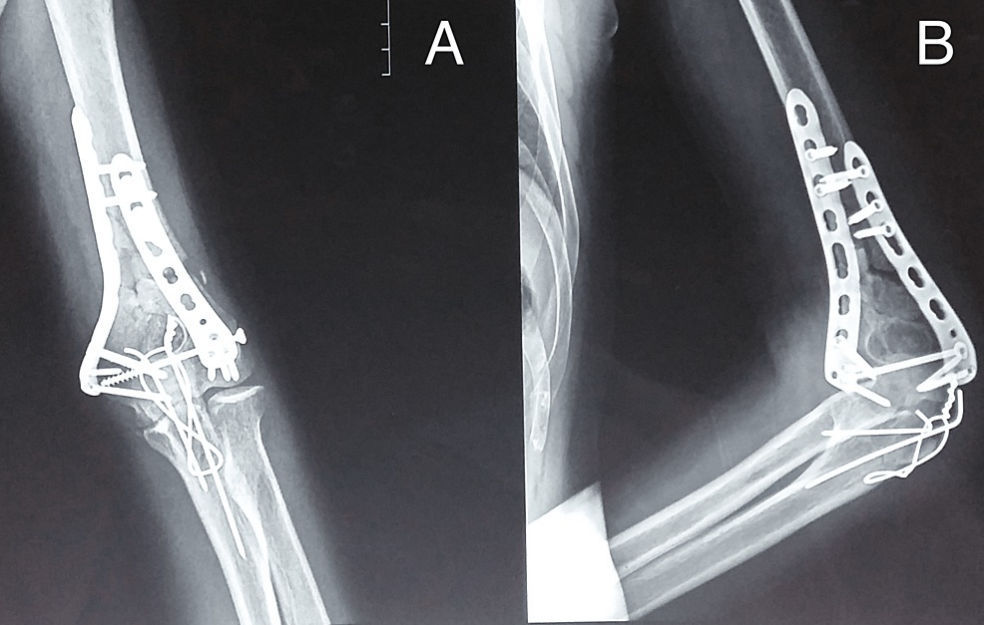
Sixth-month postoperative follow-up radiograph. The final follow-up radiograph showing fracture union. A: anteroposterior view; B: lateral view.
